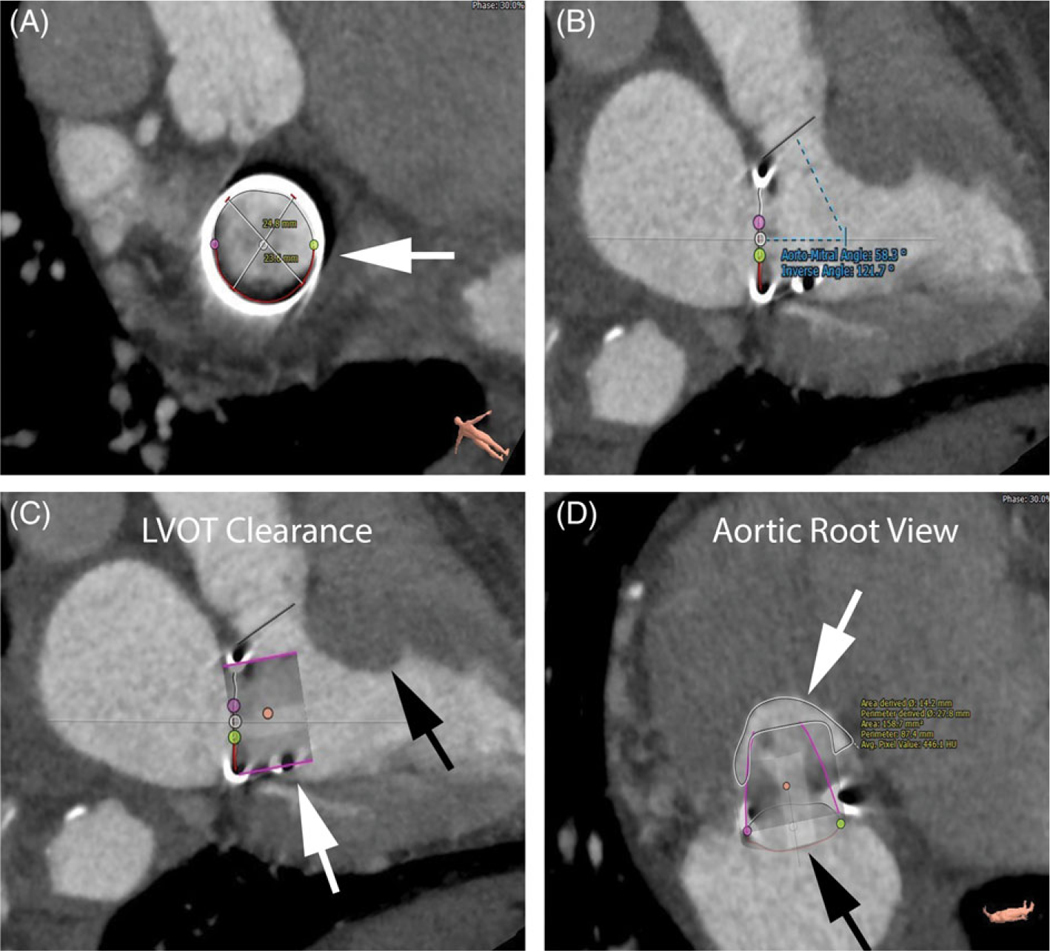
Preoperative CT modeling for case 2. A, Surgical valve frame (white
